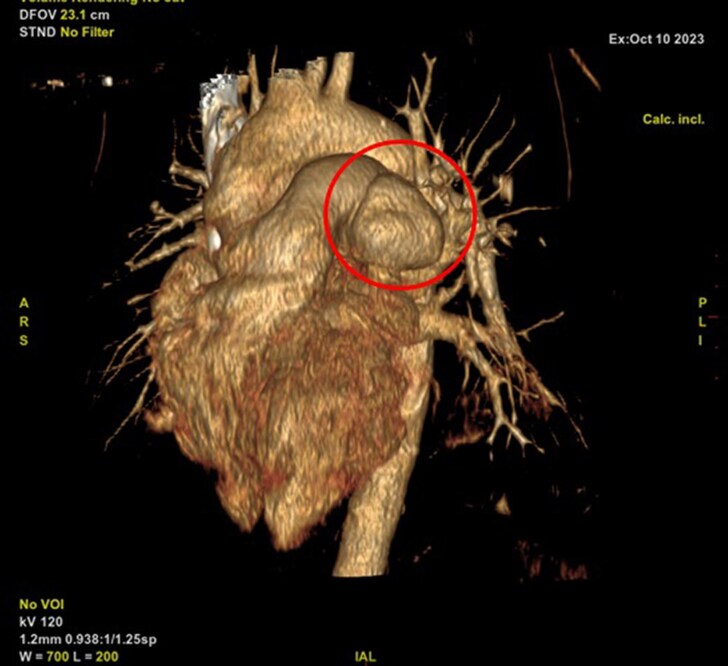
3D reconstruction of a CT scan showing aneurysms on the trunk of the pulmonary artery.
